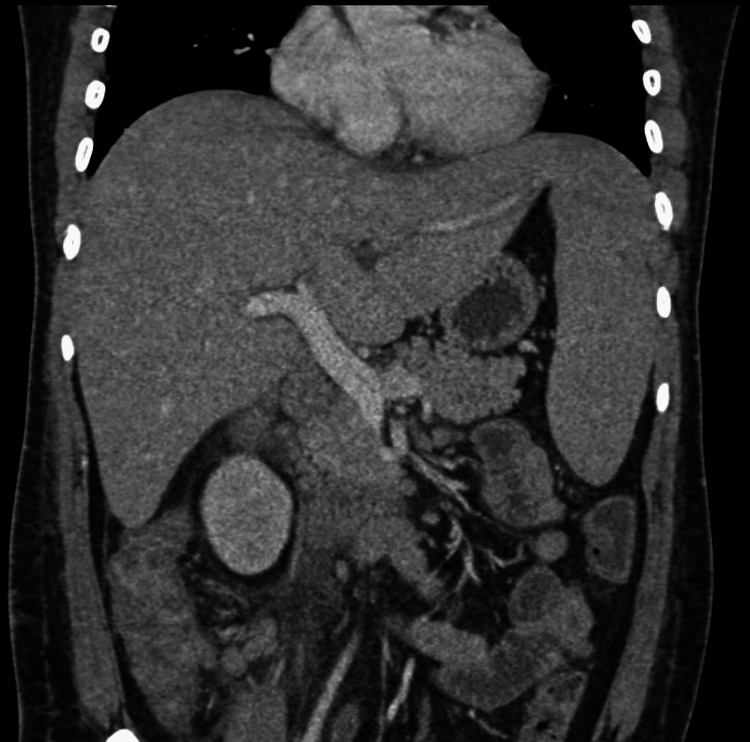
A computed tomography scan of the abdomen (coronal section) showing hepatosplenomegaly The liver was measured to be 20 cm (craniocaudal length in the midclavicular line). The spleen was measured to be 15 cm (craniocaudal length).
